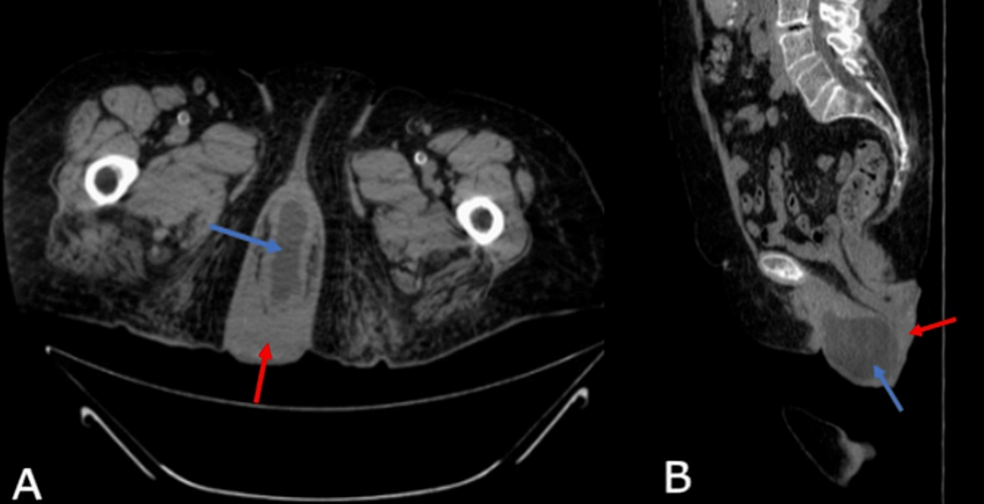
CT scans of the abdomen without contrast; axial and sagittal views. Axial and sagittal abdominal CT scans without IV contrast (A and B) demonstrating an abnormally positioned urinary bladder below the pubic symphysis (blue arrows indicative of a grade III cystocele), as well as prolapse of the uterus (red arrows).
